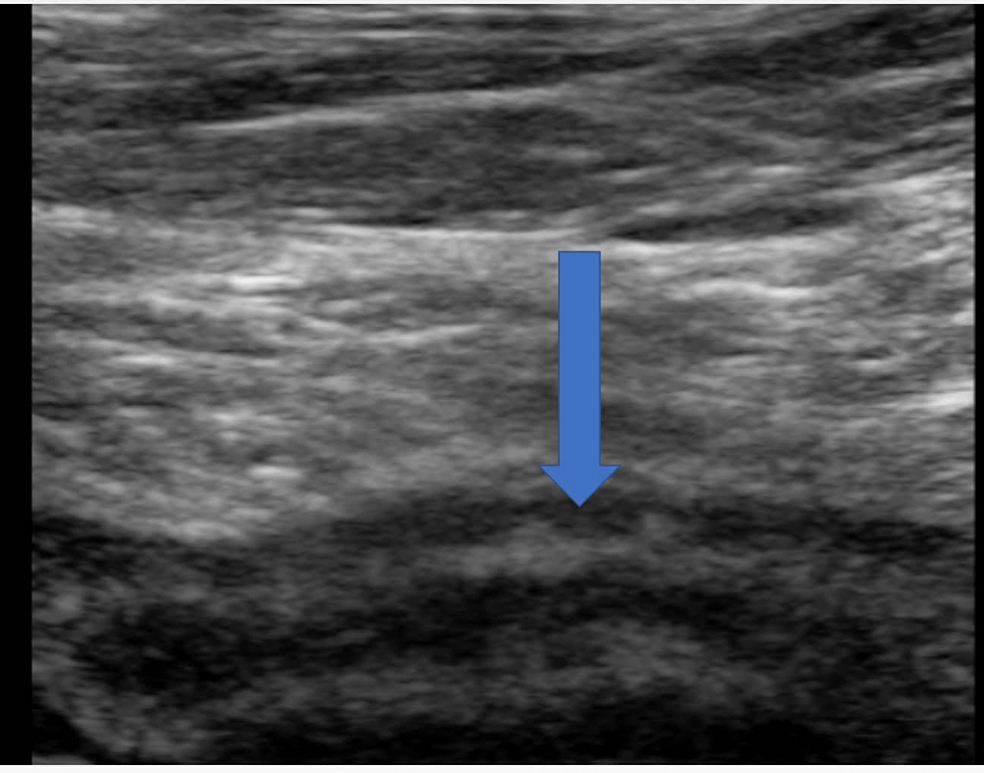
Longitudinal image of the right inguinal area ultrasound done on June 22nd.
